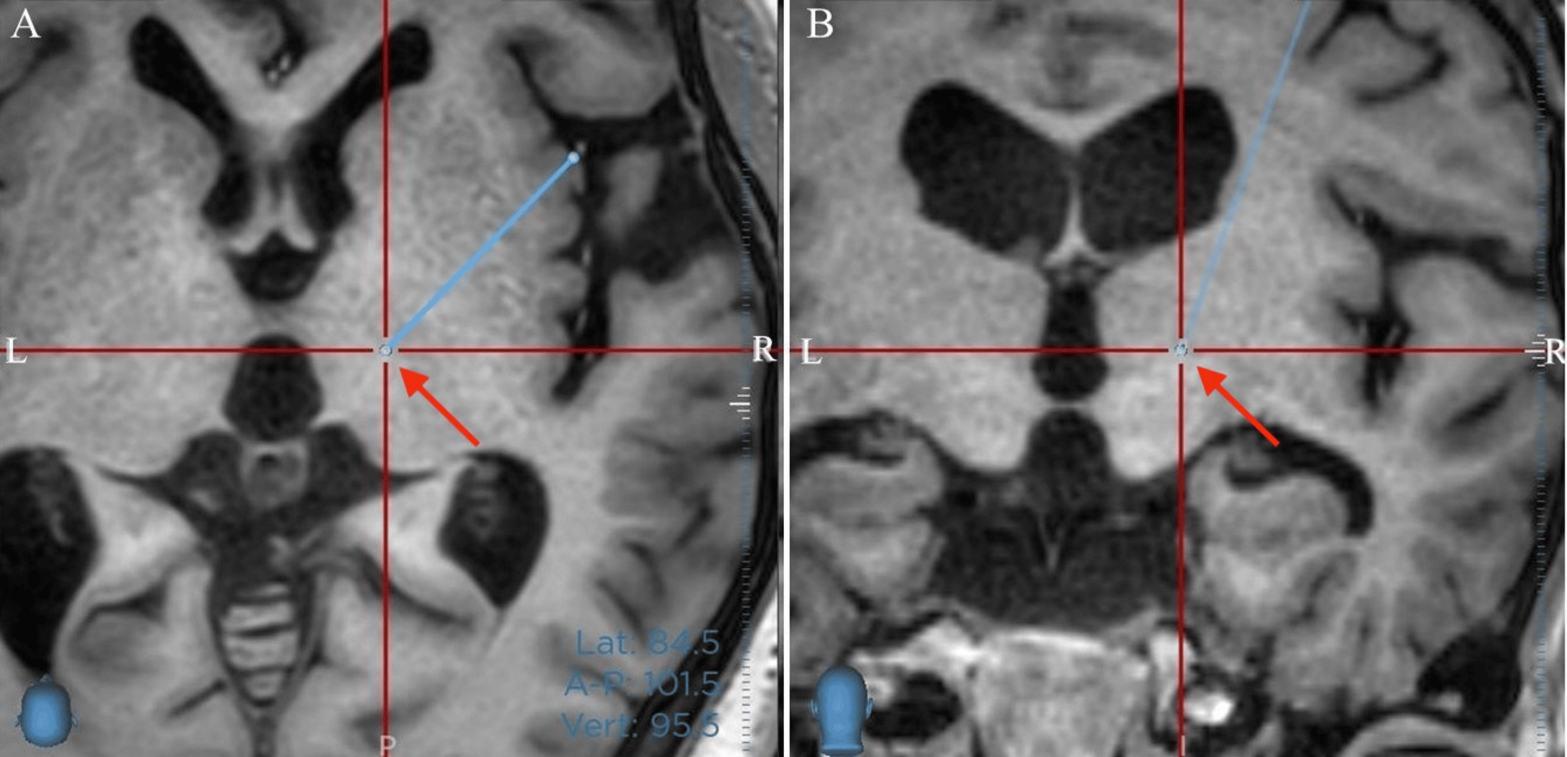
Preoperative target is in the red cross line center. A: Axial, B: Coronal sections on T1WI. Unlike a regular MRI, the screen's right side corresponds to the patient's right side. The light blue line points to the trajectory and the red arrow points to the target.
